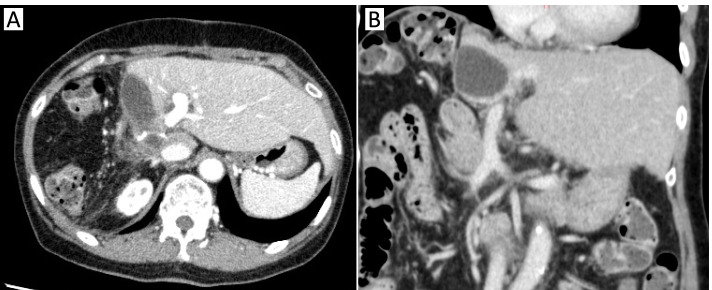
Latest enhanced CT scan done one and half years after the surgery, showing that there is no residual tumor and recurrence
